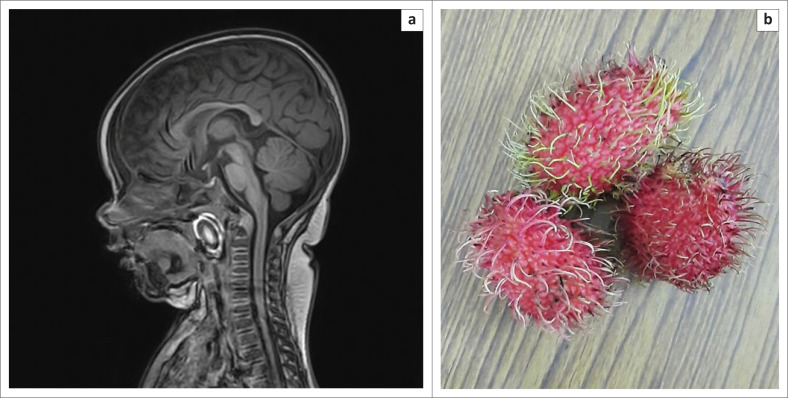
Sagittal reformatted magnetic resonance imaging brain image (a) shows the first documented case of nasopharyngeal lodgement of an aspirated rambutan fruit (b) in a 6-month-old child who was brought to the emergency room with asphyxiation and cardiac arrest. Magnetic resonance imaging brain was performed to evaluate for encephalopathy-related changes when the exotic fruit was identified in the nasopharynx.
